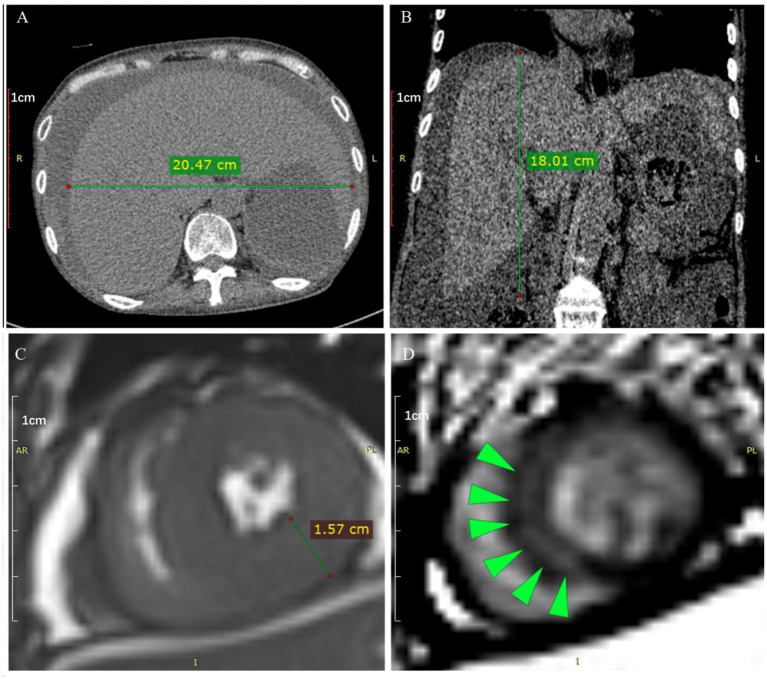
Non-contrast abdominal computed tomography (CT) scan and cardiac magnetic resonance imaging (MRI). Measurements of the transverse and craniocaudal lengths of the liver at the axial (A) and coronal (B) sections are shown. Hypertrophic myocardium, as indicated by a wall thickness of 1.57 cm at the end-systolic phase (C), and left ventricular subendocardial enhancement (D) are highlighted (green arrowheads) by phase-sensitive inversion recovery (PSIR) late gadolinium enhancement (LGE) imaging, both in the short-axis plane.
